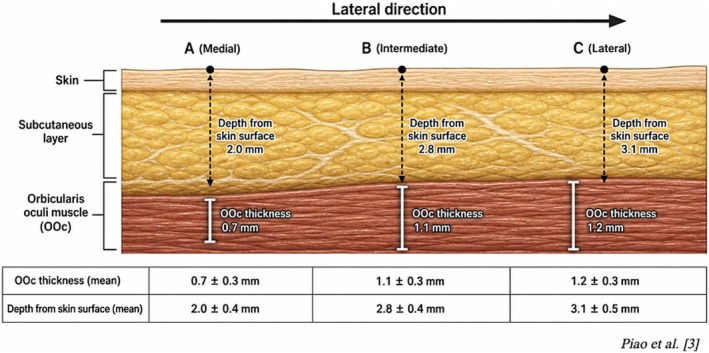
Ultrasonographic thickness gradient of the lateral orbicularis oculi muscle (OOc). Based on the ultrasound study by Piao et al. [3], the measured OOc thickness increased from point A to point C: 0.7 ± 0.3 mm at A, 1.1 ± 0.3 mm at B, and 1.2 ± 0.3 mm at C. The corresponding depths from skin surface were 2.0 ± 0.4 mm, 2.8 ± 0.4 mm, and 3.1 ± 0.5 mm. In precise wording, the more lateral measured portion at the lateral canthal level was thicker than the more medial measured portion, rather than the entire lateral OOc being uniformly thicker than the entire medial OOc. Image regenerated in courtesy of Prof. Hee Jin Kim.
